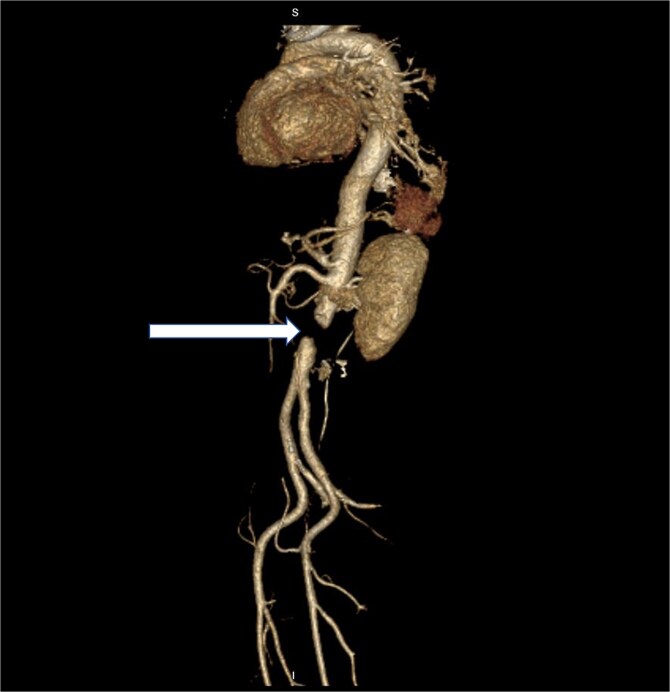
3D reconstructed postoperative CT scan of the abdomen with the arrow showing complete aortic occlusion.
